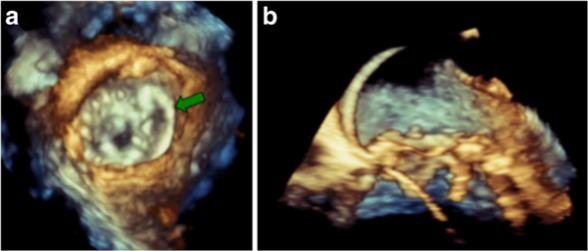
Real-time 3-D Trans-esophageal echocardiography. 3-D Zoom view on 3-D TEE pre-deployment showing (a) antero-medial paravalvular defect in the mitral valve (*green arrow*) and (b) wire passing through paravalvular leak
